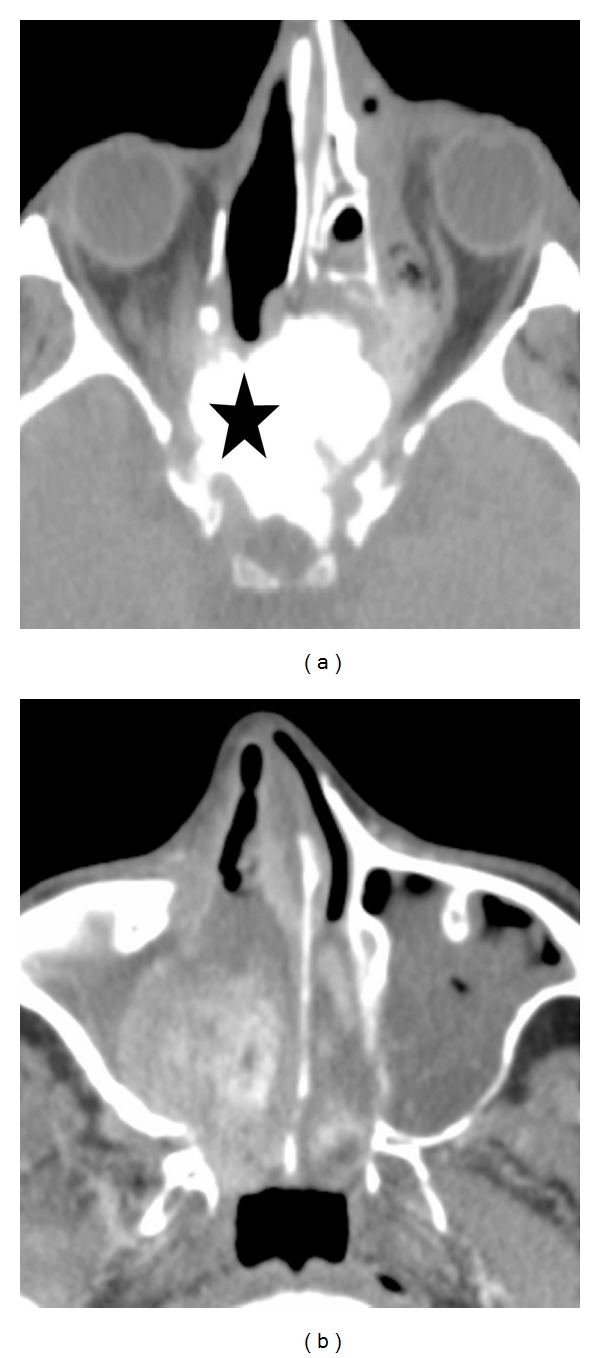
(a) Initial facial axial CT scan showing a mixed iso- and hyperdense mass (black star) of the right lateral nasal wall extending to the left orbit, (b) facial axial CT scan one month later showing progression of the tumor to involve the right maxillary sinus, the nasal fossa.
